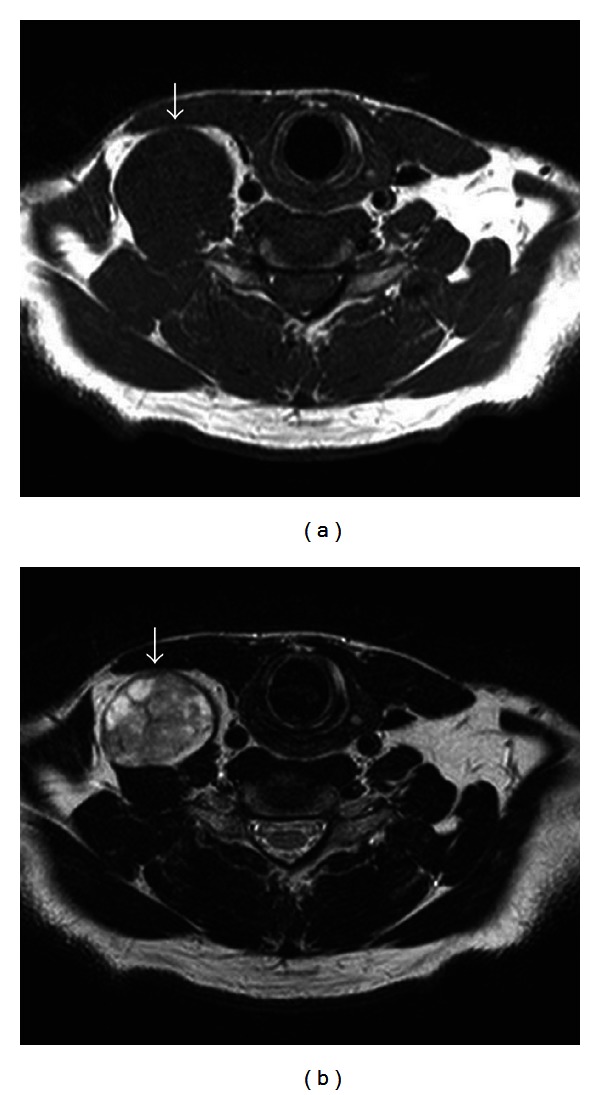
MRI findings for case 24. (a) Axial T1-weighted imaging showed a mass with signal hypointensity (arrow). (b) Axial T2-weighted imaging showed a mass with heterogeneous signal hyperintensity (arrow).
